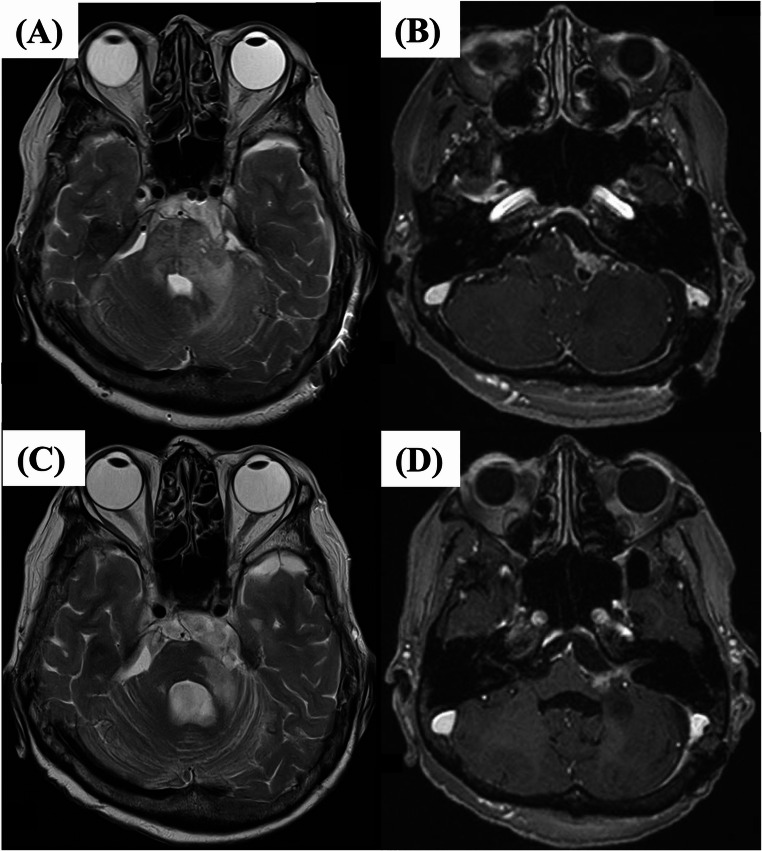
Postoperative magnetic resonance imaging (MRI). T2-weighted (a) and T1-weighted imaging with gadolinium enhancement (b) showing the residual tumor with high-intensity signal at the middle cerebellar peduncle (a) and tumor along the facial nerve (b). MRI after radiotherapy. T2- (c) and T1-weighted imaging with gadolinium enhancement (d) show regression of the perifocal edema (c) and residual tumor (d)
